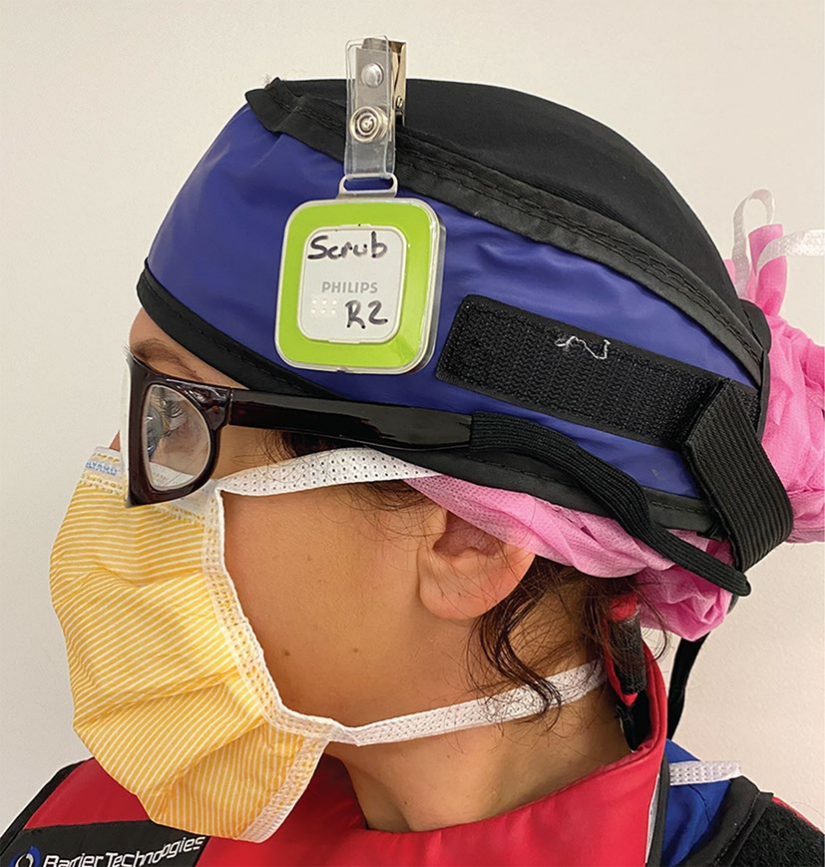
Location of DoseAware badge when attached external to skull cap. The dosimeter badge was worn on the temple closest to the x-ray tube gantry
